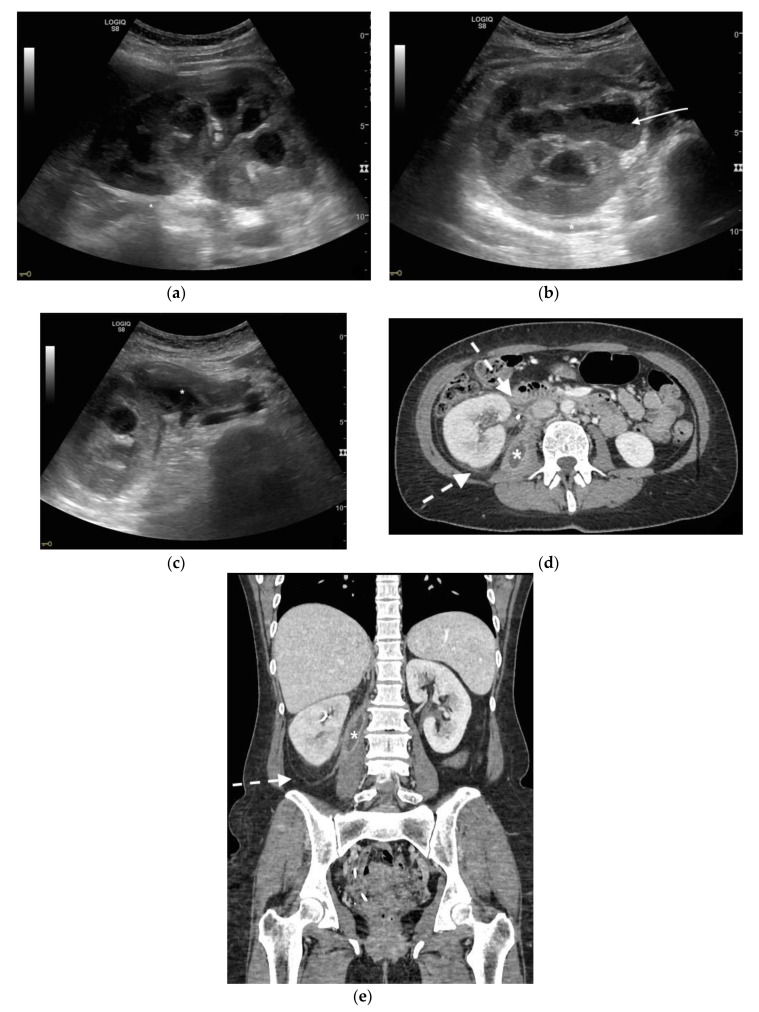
A 48 years old septic woman. US longitudinal (a) and axial (b,c) view of the right kidney. Enlargement of the kidney, hydronephrosis with sharply defined urine-debris level (white arrow). Thickening of perirenal fascia and markedly inhomogeneous echogenicity of perirenal fat suggesting extrarenal extension (*). The patient underwent nephrostomy in the emergency setting, and CT was performed after stent placement. Axial (d) and MPR coronal (e) CT with intravenous contrast in parenchymal phase, showing correct stent positioning (white arrow) and drainage of calyceal-pelvic system. The right kidney appeared enlarged with a delayed parenchymal phase, the calyceal-pelvic system was unstretched after nephrostomy placement. Right perirenal fascia was thickened posteriorly (dashed arrow) and extrarenal extension of the pathology was confirmed by the presence of an abscess in the right iliopsoas muscle (*).
